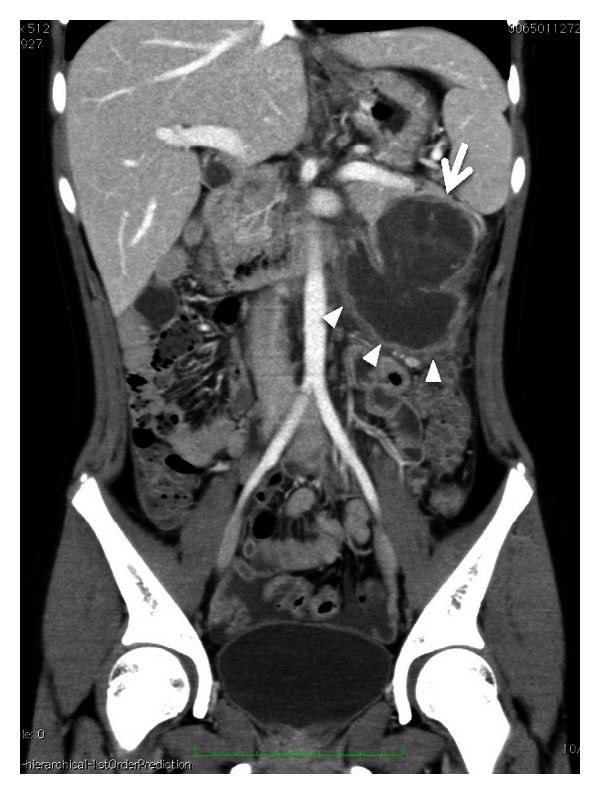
Urgent CT of acute abdomen. A cystic tumor is shown (arrow) in the pancreatic tail and fluid collection (arrowhead) below it. CT also revealed fluid retention in the pelvis. These findings suggested the rupture of tumor and hemoperitoneum.
